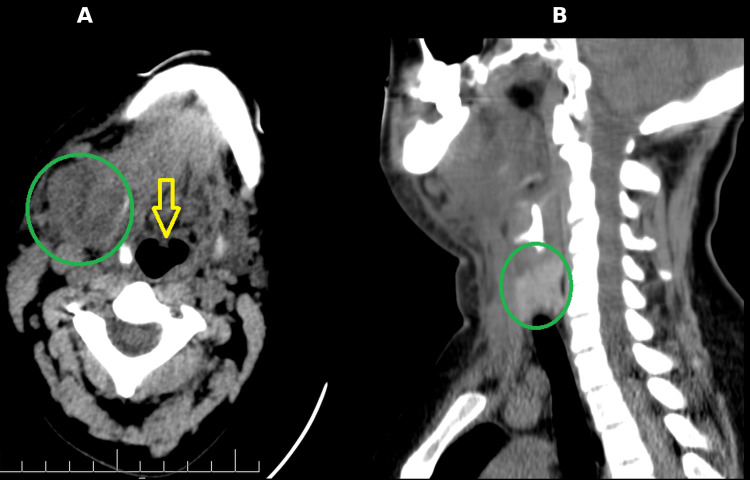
Preoperative CT Neck Figure 1A (axial): Green circle - right submandibular inflammatory mass and collection medial to the right submandibular gland with sternocleidomastoid involvement. Yellow arrow - clinician-confirmed leftward tracheal deviation. Figure 1B (sagittal): Green circle - right-sided deep neck space collection within the sternocleidomastoid region. All images are fully anonymised; patient identifiers, accession numbers, timestamps, and provider names have been removed.
